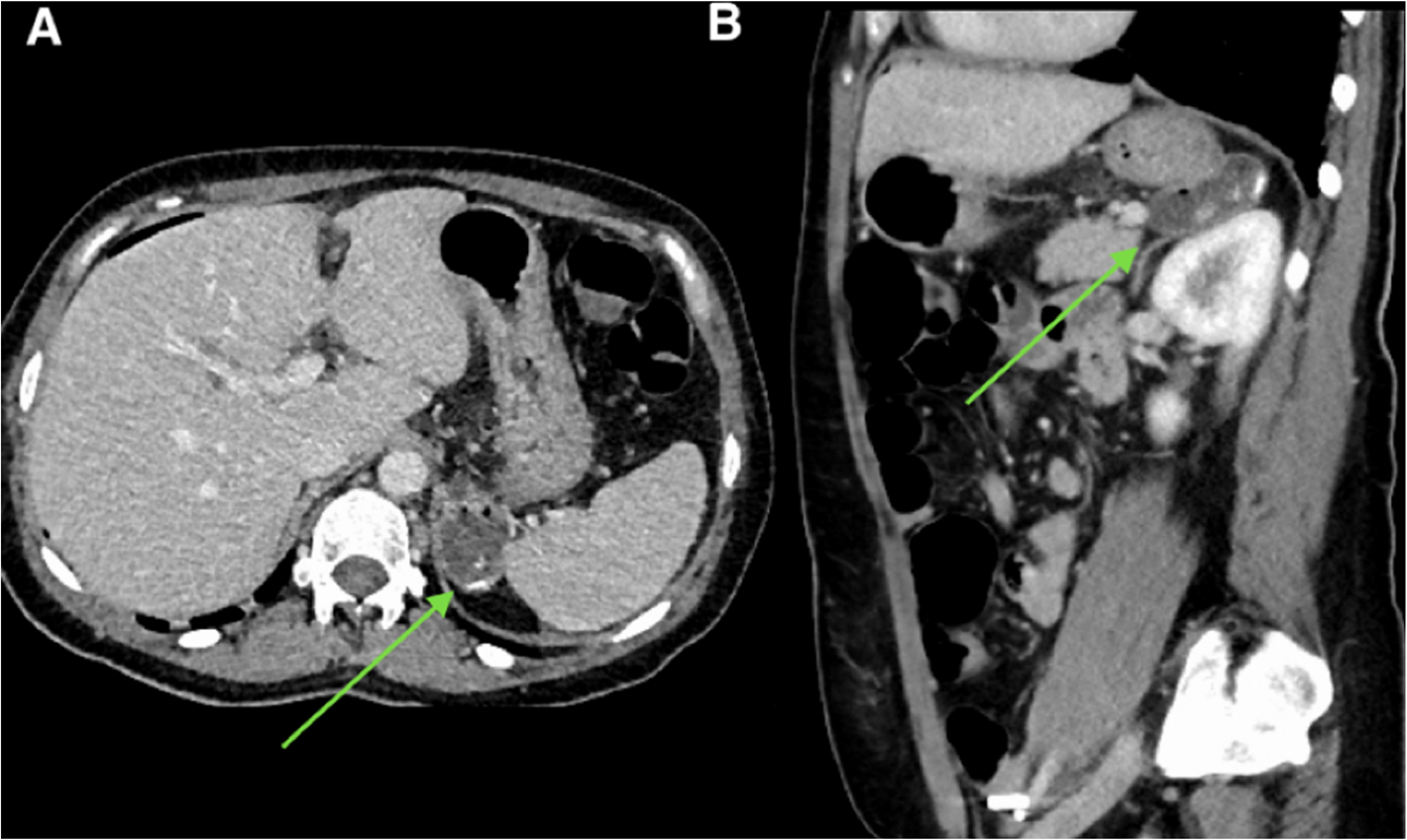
Axial and sagittal images of a contrast-enhanced CT abdomen in a patient several days post-laparoscopic cholecystectomy. There is a rim-enhancing fluid collection compatible with an abscess which contains multiple (dropped) gallstones
